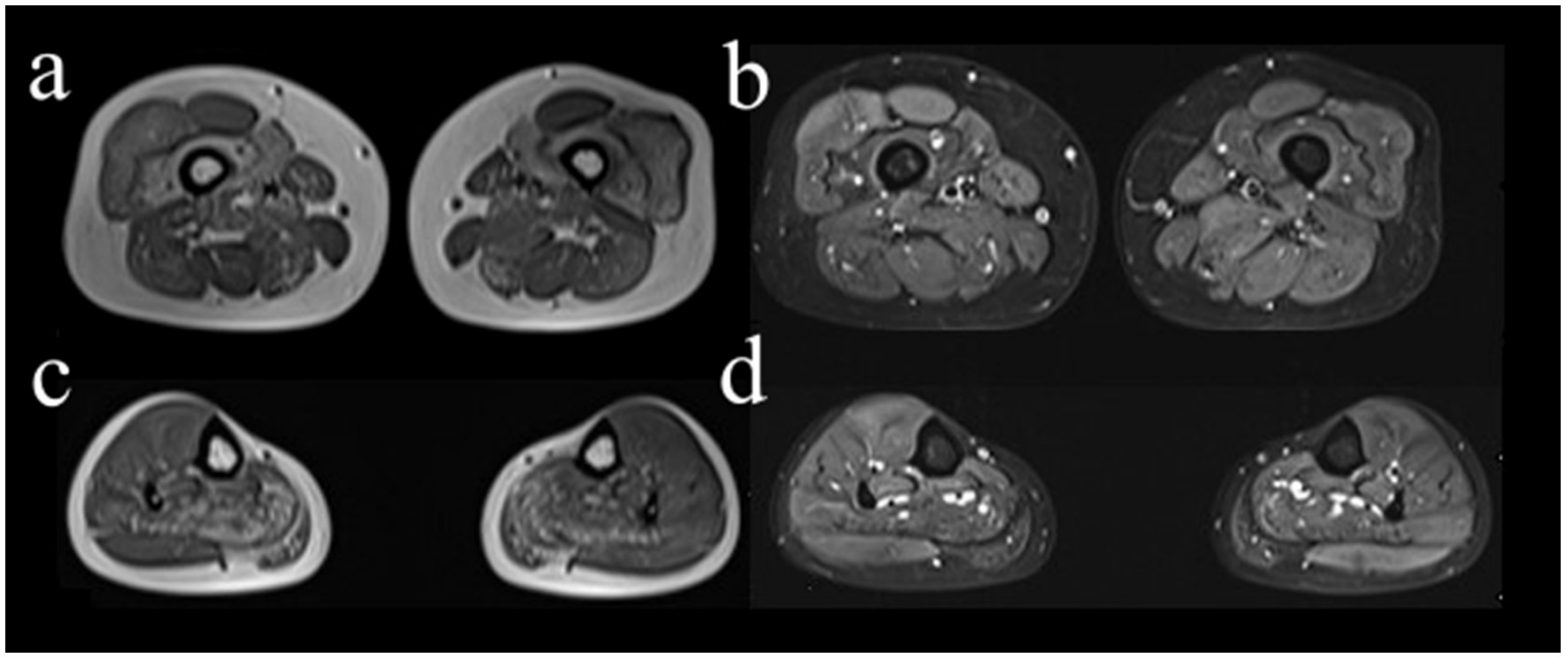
Axial MRI images of right lower limb muscles. The right vastus intermedius muscle (a, b), medial head of gastrocnemius muscle, and soleus muscle (c, d) showed spotted fatty degeneration. The MRI sequences were T1-weighted imaging (a, c) and fat-suppressed T2-weighted imaging (b, d), respectively.
